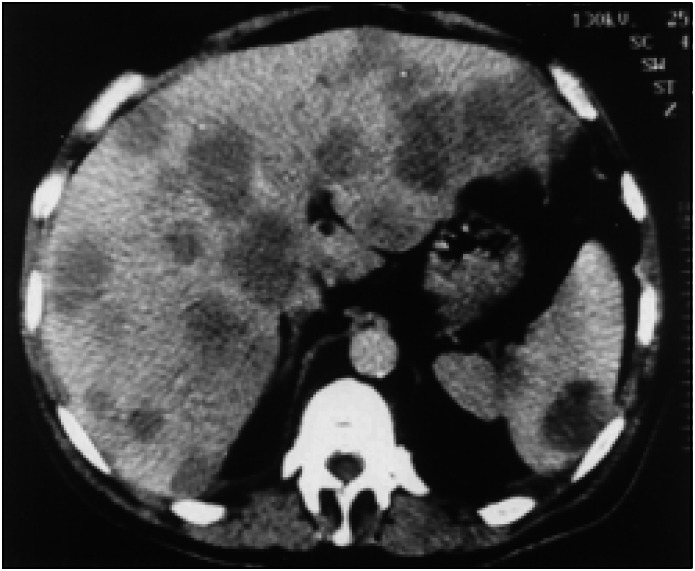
Abdominal computed tomography, showing widespread hypodense nodules in the hepatic and spleen beds, corresponding to a metastatic neoplasm.
